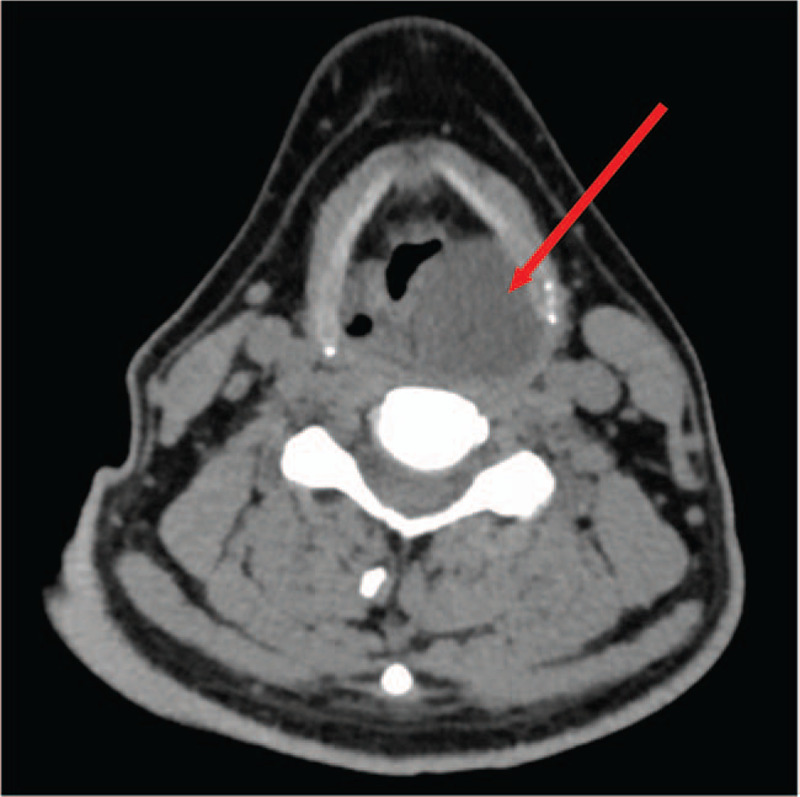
A laryngeal enhanced CT revealed a space occupying lesion of approximately 4.5cm × 3.0 cm × 2.0 cm in size located in postcricoid area.
